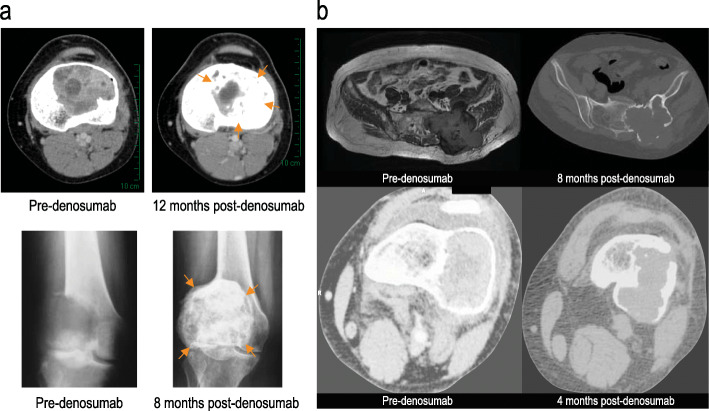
Misdiagnosis of GCTB. a Typical response of GCTB to denosumab leads to bone formation and calcification (top two images are axial CT soft tissue window and bottom two images are anteroposterior radiographs). b In misdiagnosed PMGCTB, poor calcification in response to denosumab is shown (top two images are axial T1-weighted MRI, axial CT bone windows and bottom two are axial CT soft tissue windows)
